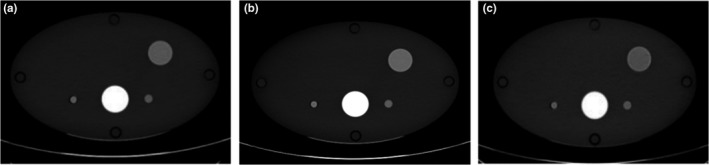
Example of images used for measuring enhancement in intermediately enhancing lesions (a) true unenhanced image (b) 70‐keV monochromatic image reconstructed from the Dual‐Energy post‐contrast dataset (c) virtual unenhanced image reconstructed from Dual‐Energy computed tomography dataset.
